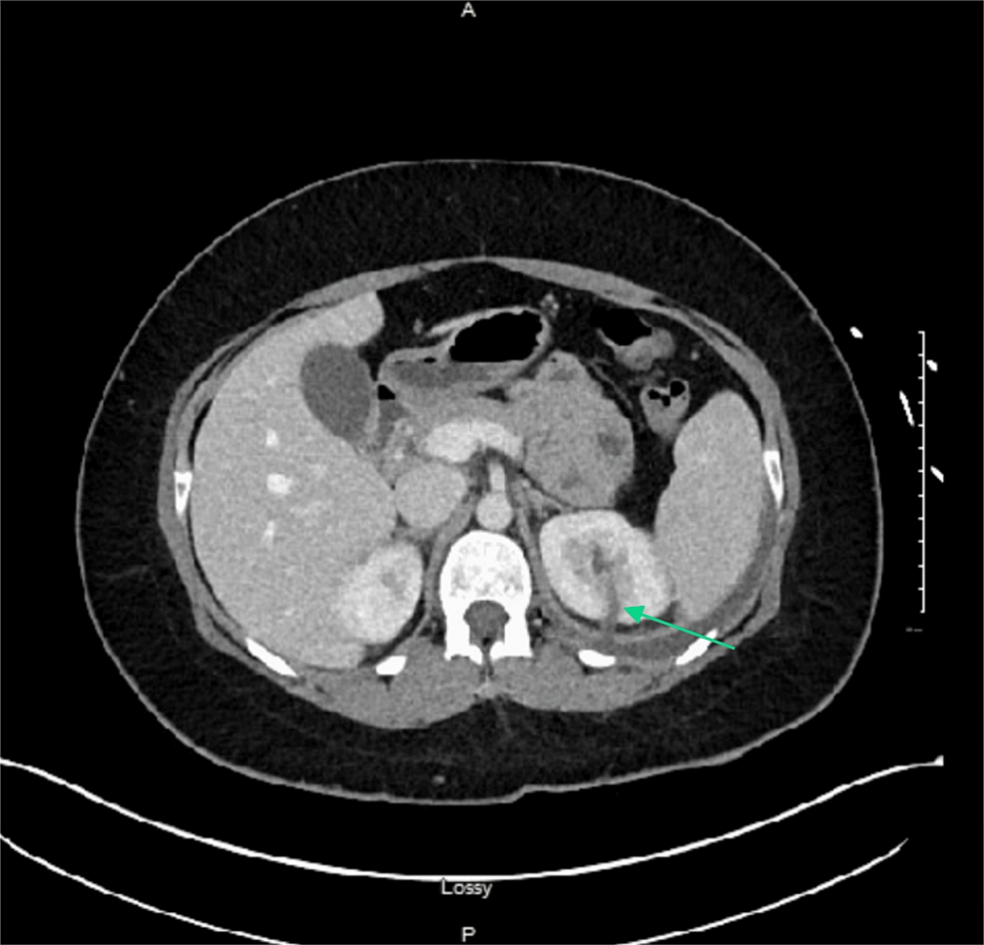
CT abdomen/pelvis with contrast. The green arrow indicates a posterior defect in the left kidney
